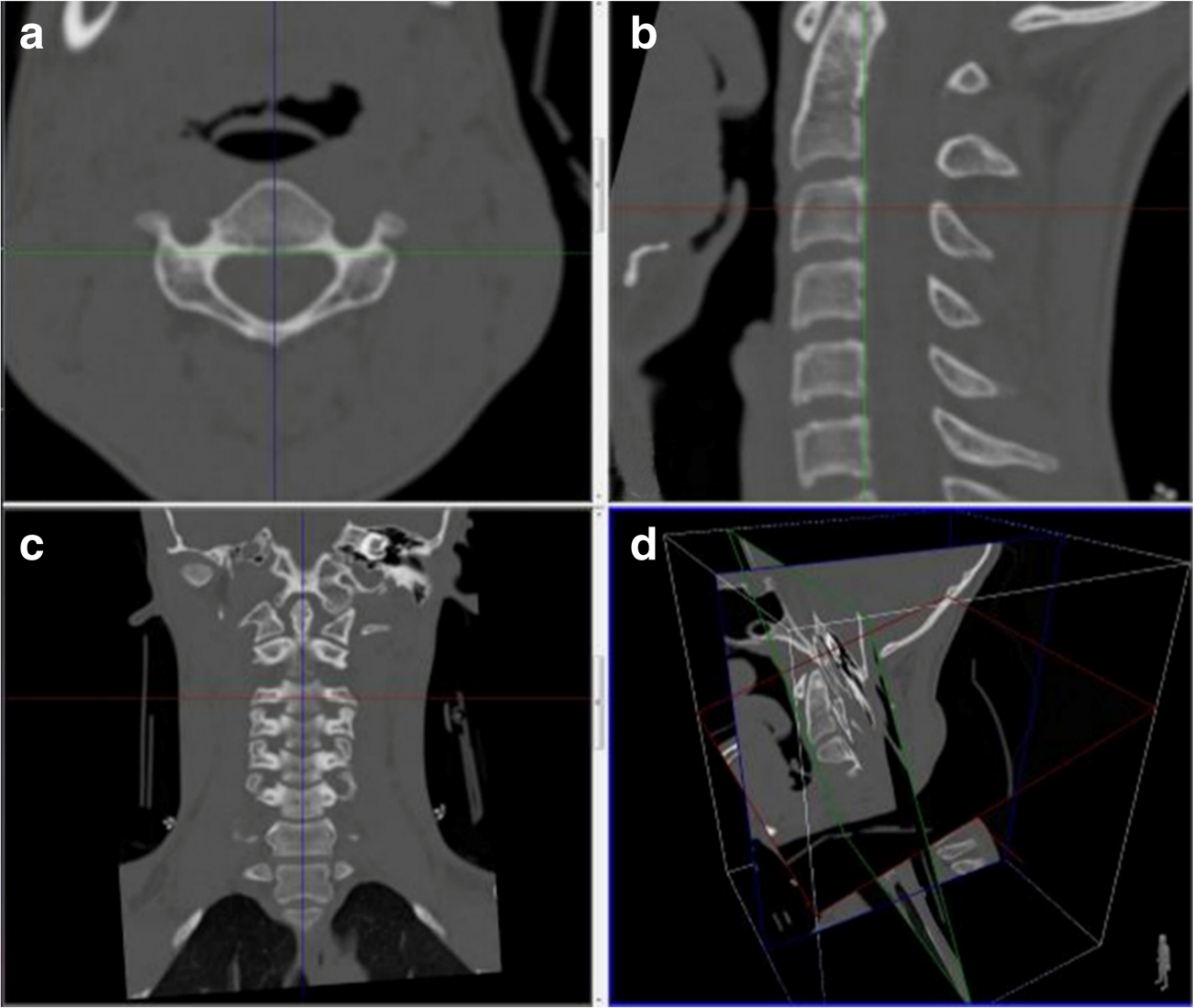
Determination of the CAP and CTR with axial (a), sagittal (b), coronal (c), and three-dimensional projections of section plans (d) reconstructed CT images
